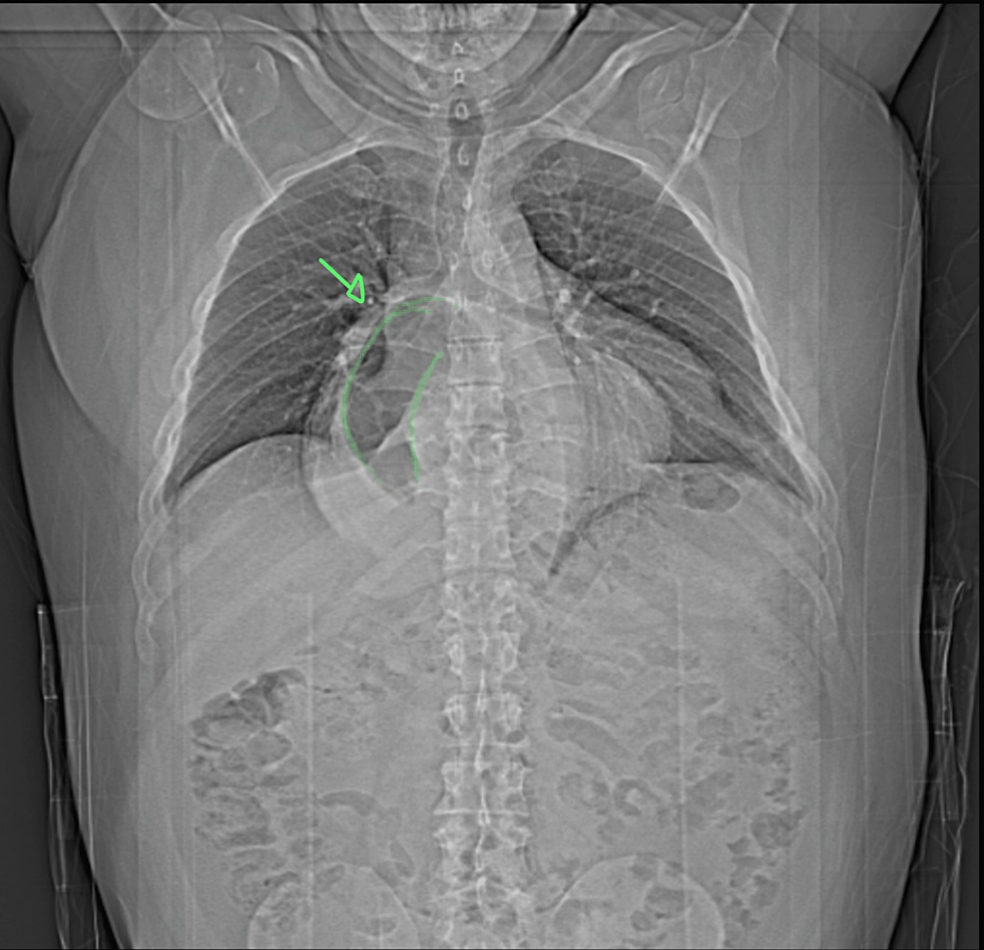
Preoperative anterior-posterior chest X-ray The green arrow and green outline indicate the stomach.
